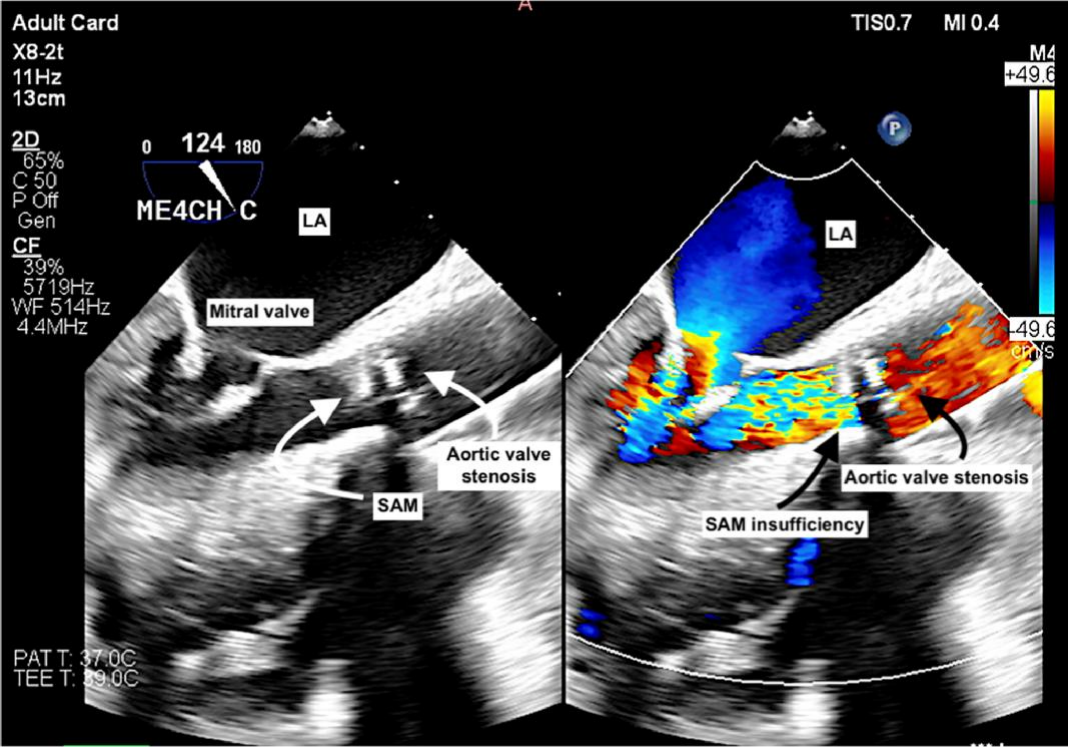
Transoesophageal echocardiography with and without colour Doppler demonstrating the presence of a subaortic membrane causing regurgitant blood flow through the aortic valve.
